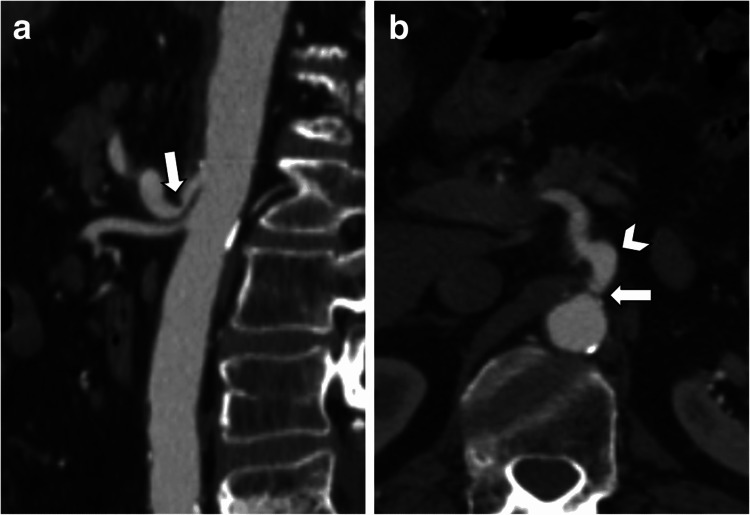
CT scan of celiac artery compression by the median arcuate ligament. (a) Sagittal view showing severe stenosis at celiac artery origin without arthrosclerosis; (b) axial view of celiac artery compression in the presence of post-stenotic dilation. The figure is taken from Gozzo et al. [1]. This article is licensed under a Creative Commons Attribution 4.0 International License, which permits use, sharing, adaptation, distribution, and reproduction in any medium or format, as long as you give appropriate credit to the original author(s) and the source (http://creativecommons.org/licenses/by/4.0/). CT: computed tomography.
